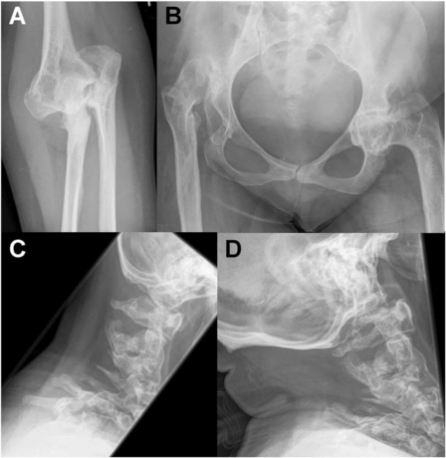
Radiographs of patient 6. Dislocation of the right elbow: olecranon dislocated to the ulnar side without articulation (a). Right side of the hip AP: cranial dislocated and ossified with neojoint X; left side: varus position with arthrotic joint, sclerotic fringe and small intra-articular space and spinoiliacal osteophyte (b). Functional graphs of the cervical spine did not provide evidence for cervical instability but showed deformed cervical vertebral bodies with fusion C3/C4(c and d). AP: anteroposterior
